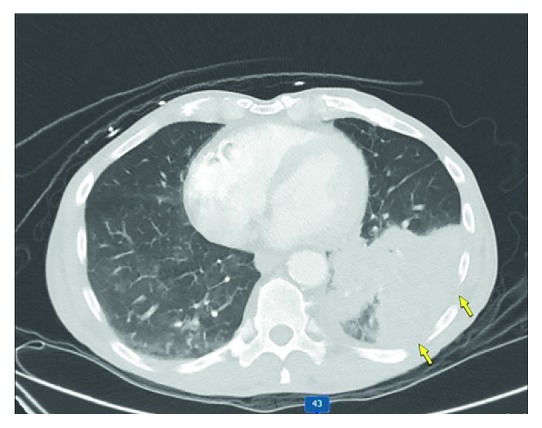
CT chest demonstrating multifocal pneumonia in the left lung base with areas of suspected internal necrosis. No mediastinal lymphadenopathy is visible.
